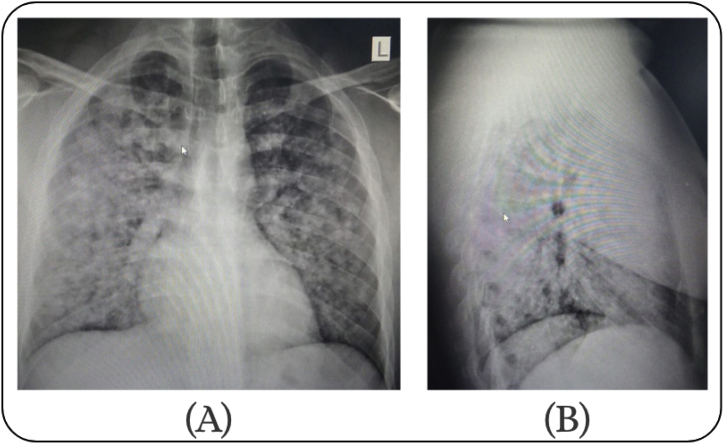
Posteroanterior and lateral Chest X-ray [A,B] images show diffuse bilateral hazy opacities, predominantly in the middle and lower zones, mostly suggestive of a widespread alveolar or interstitial process.
